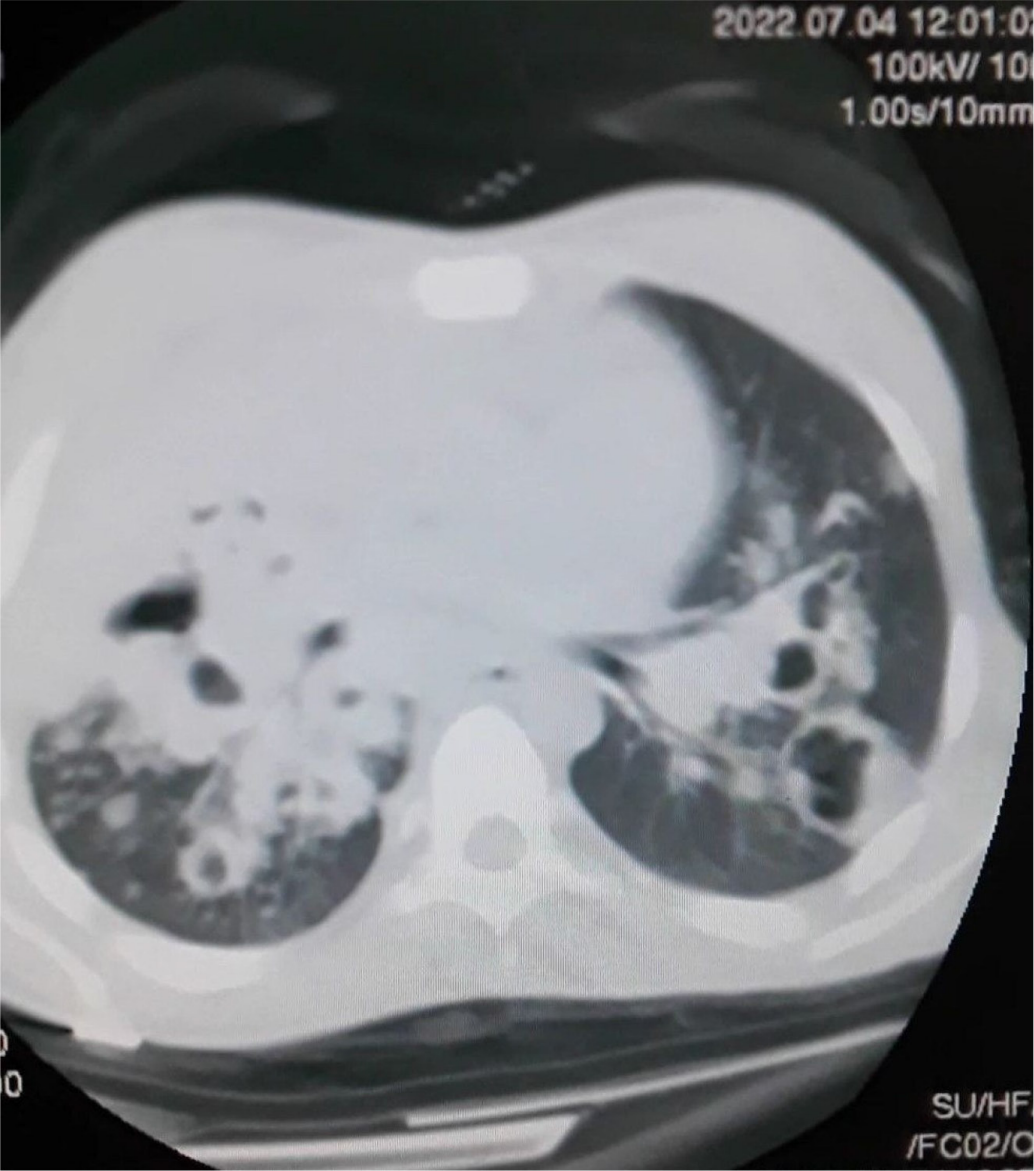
Computed tomography (CT) scan demonstrated density, infiltrates, and cavities in the lungs.
